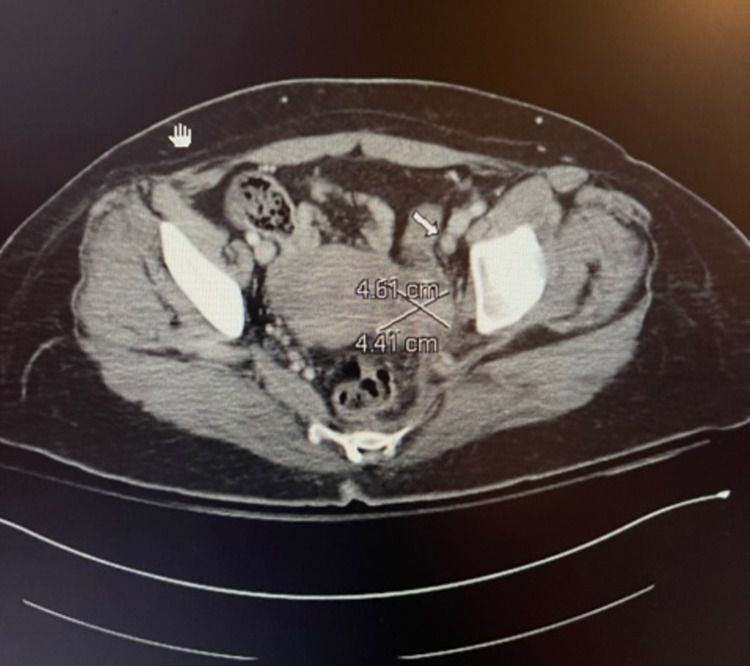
CT abdomen and pelvis on admission Left adnexa mass is 4.61 x 4.41 cm in dimension; left side ureter is compressed by the mass posteriorly (white arrow) CT: computed tomography
